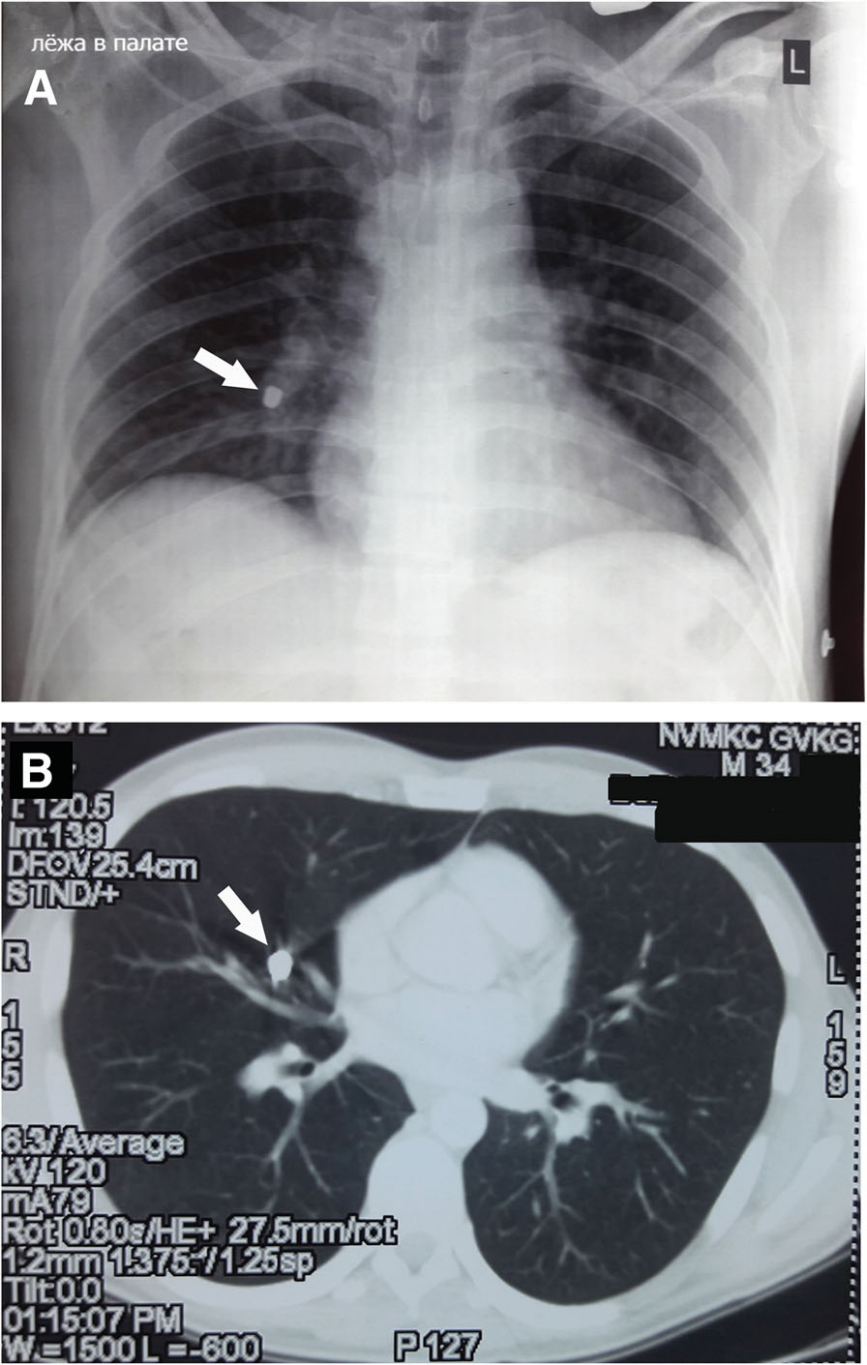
Visualization of the projectile-embolus (*marked with an arrow*) in the right mid-lobe pulmonary artery on the 63rd day after the injury by chest X-ray (a) and by spiral computed tomography (b)
